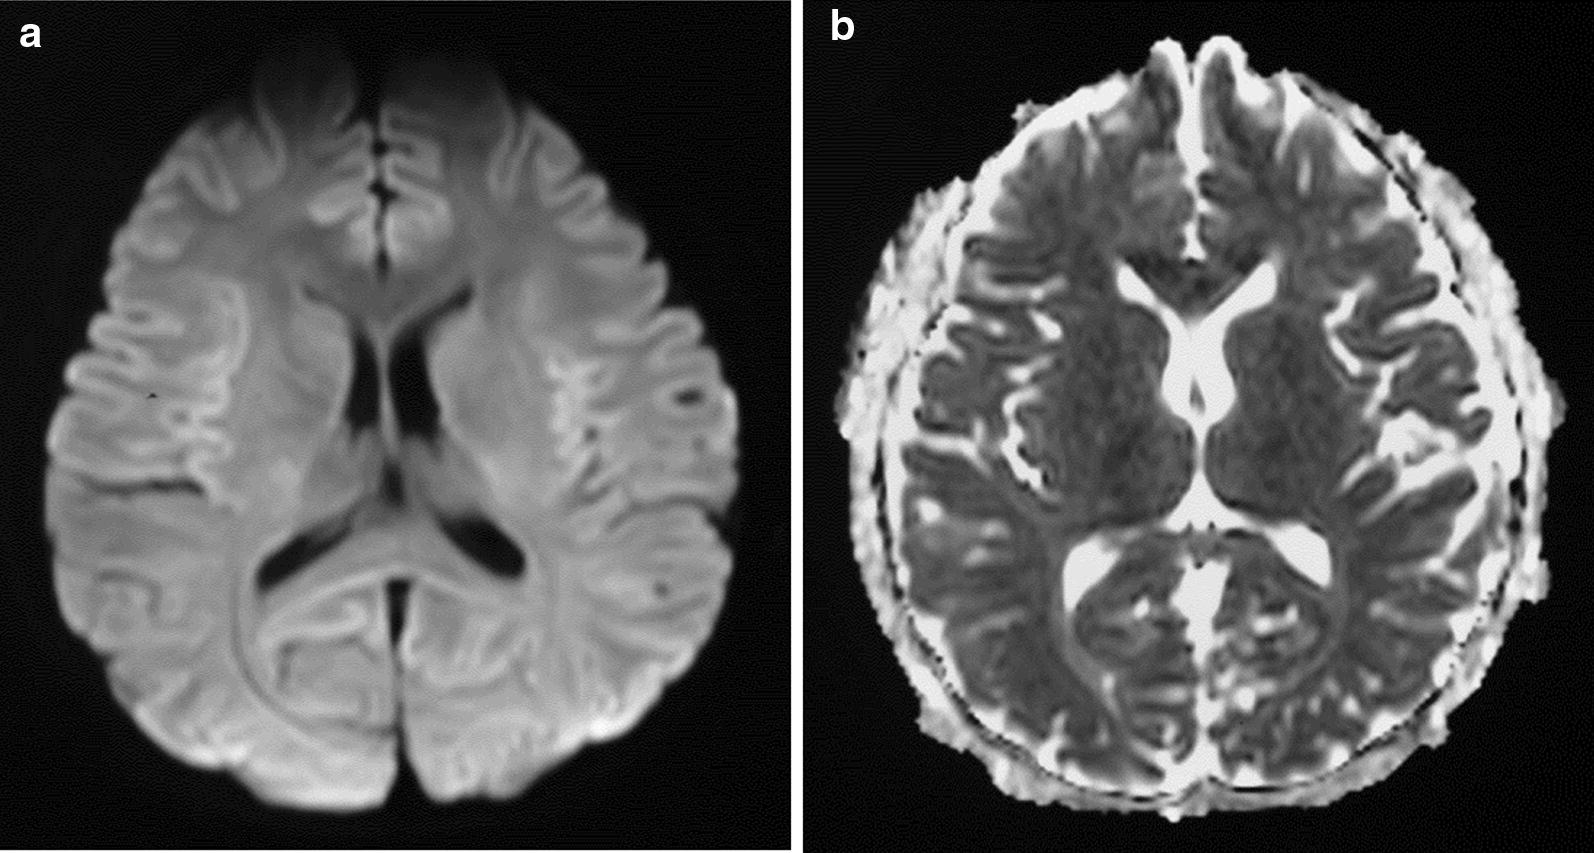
Follow-up MRI brain (a) and diffusion-weighted imaging (b) after 1 month showed resolution of the lesion
